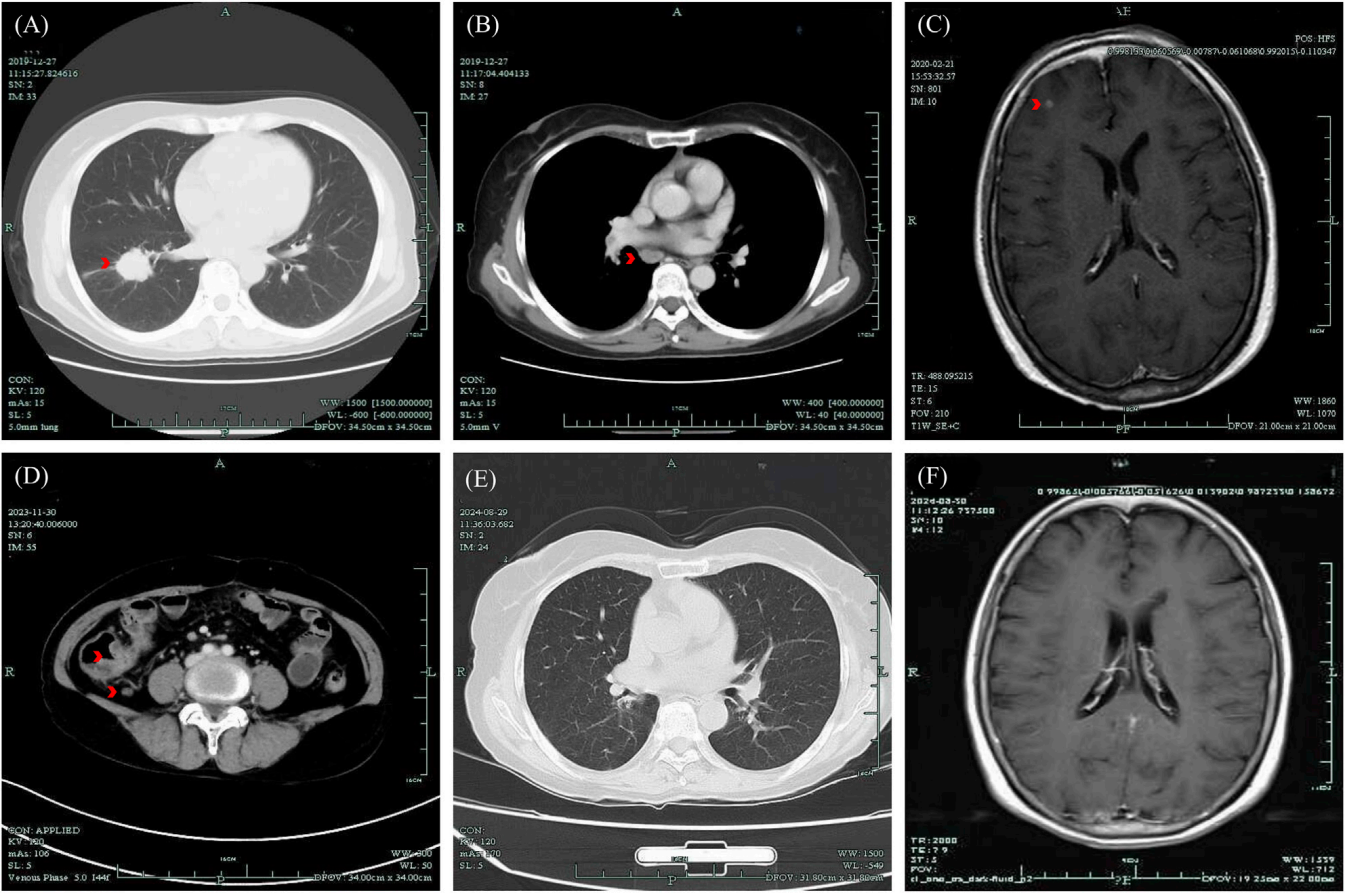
Medical images presented based on the clinical course of the patient. (A, B) CT image of lung lesion and enlarged mediastinal lymph nodes, December 2019; (C) MR image of brain metastases found post-operatively, February 2020; (D) CT image of thickening of the ileocecal junction, December 2023; (E) CT image of the chest with no tumor recurrence, 29 August 2024; (F) MR image of persistent complete remission of the intracranial lesion, August 29, 2024. CT, Computed Tomography; MR, Magnetic Resonance.
